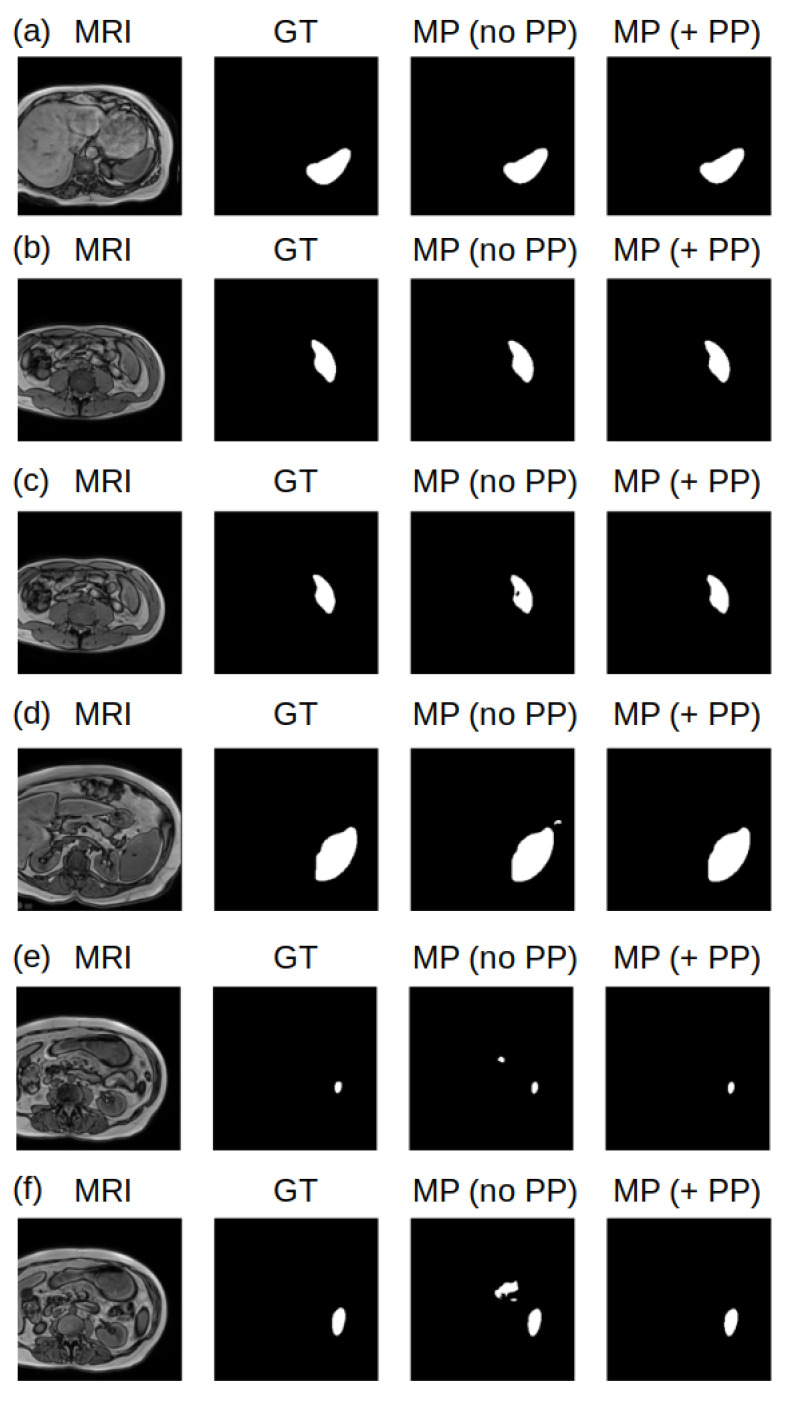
Examples of MRI slices (first column), their GT (second column), the MP (no PP) (third column), and the MP (+PP) (fourth column) for valid MP cases (a,b), filling holes (c), and removing inaccurate predictions (d–f).
